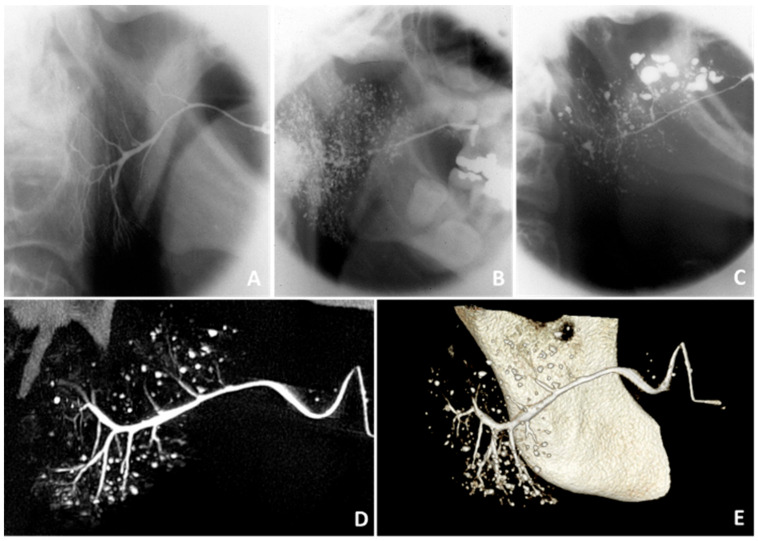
Findings on sialography. Sialographies of the parotid gland showing (A) no abnormalities in a healthy subject, (B) punctate/globular sialectasis in a pSS patient, and (C) globular/cavitary sialectasis in a pSS patient [29]. (D) Two-dimensional sialo-CBCT image and (E) three-dimensional sialo-CBCT image of the parotid gland of a pSS patient, showing normal width of the primary duct, moderate scarcity of ductal branches, and numerous diverse sialectasis. Thanks to Prof. D.J. Aframian and Dr. C. Nadler and colleagues who provided the sialo-CBCT images.
